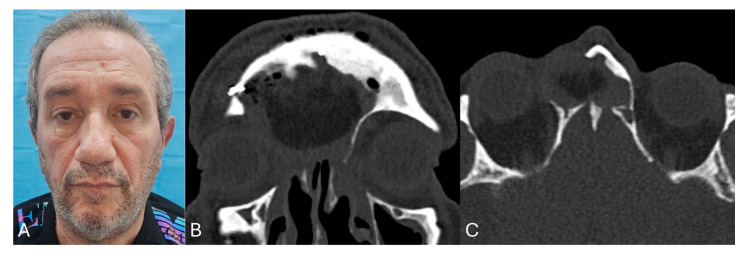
Case example post-surgery. (A) Clinical photo with evidence of resolution of dystopia and globe dislocation; (B) post-operative CT coronal view and (C) post-operative CT axial view.
